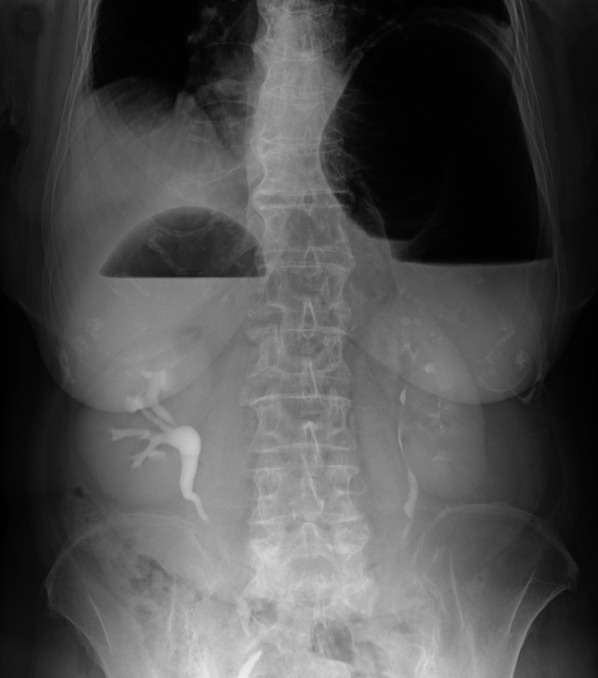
Erect chest and abdomen X-ray showed a double air-fluid level
